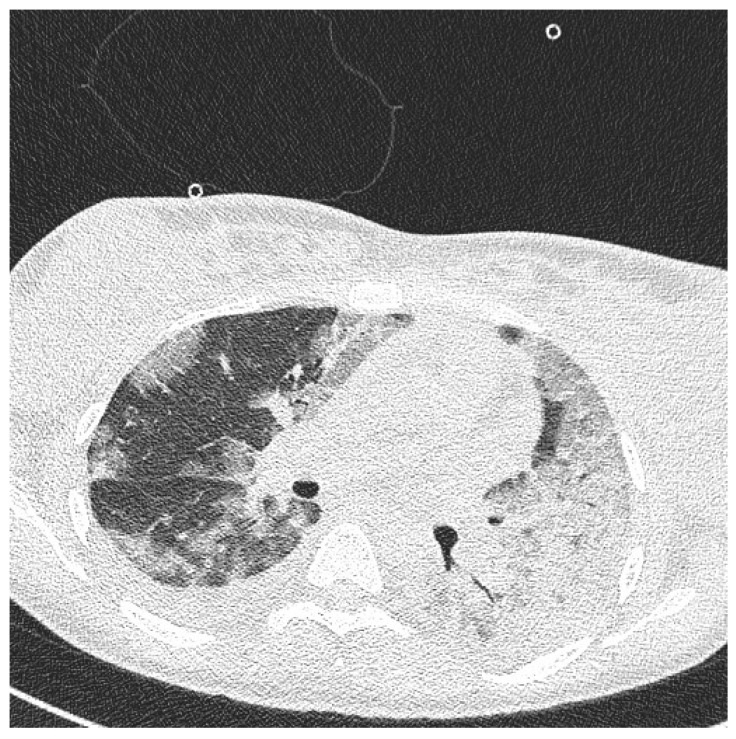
Chest Computed Tomography (HRCT).
